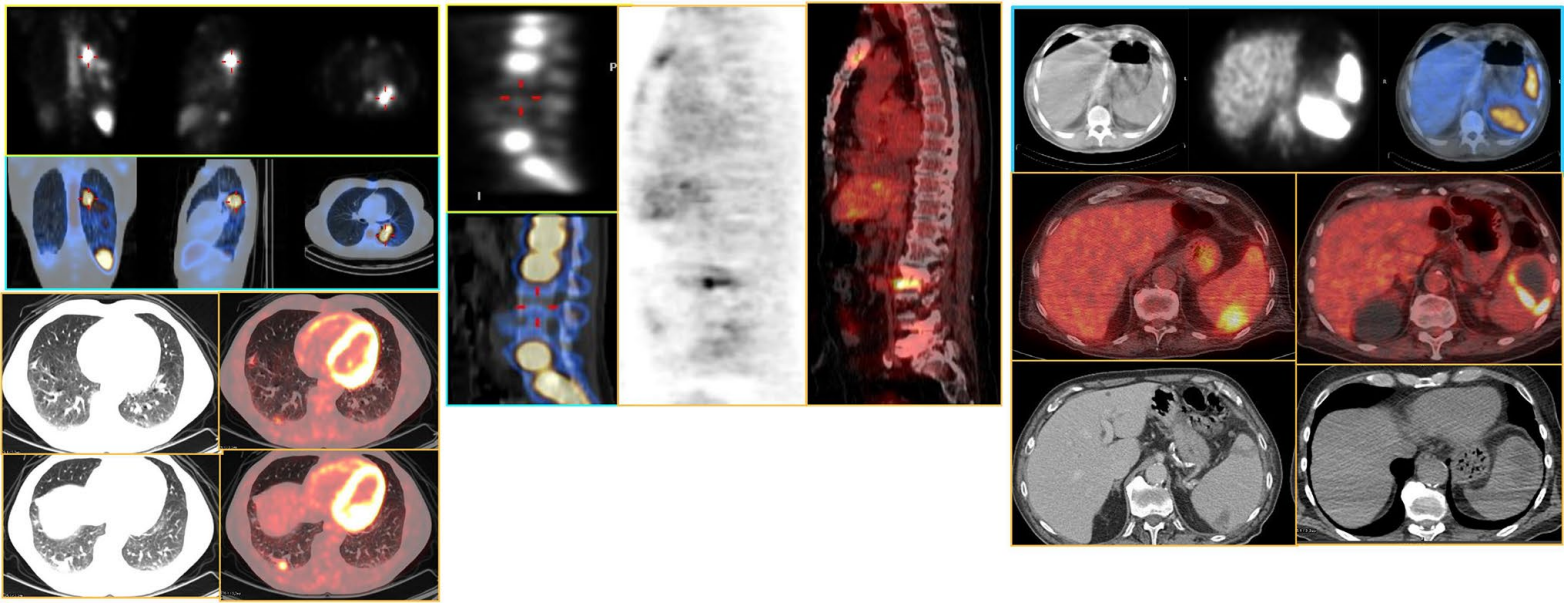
Comparison of WBC and [^18^F]FDG pattern of uptake in embolisms localized in the lung in a patient with right-sided IE appearing. In both cases, there is an area of increase radiopharmaceutical uptake (right panel WBC SPECT/CT in the upper panel in the emission and superimposed coronal, sagittal, and transaxial views, respectively, and in the lower panel [^18^F]FDG PET/CT in the transaxial CT and superimposed images at different levels). In the spine, a different pattern is seen at WBC imaging where typical cold spot is evident due to the normal high uptake of radiolabeled WBC in the normal bone marrow. On the contrary, [^18^F]FDG PET/CT finding shows increased uptake with linear pattern involving the intravertebral space, the lower aspect of the upper vertebral body, and the upper aspect of the lower vertebral body (middle panel, from right to left coronal view of WBC upper panel emission and lower panel superimposed SPECT/CT; emission and superimposed coronal [^18^F]FDG PET/CT). Embolism at spleen also presents a different uptake pattern in WBC images where photopenic area of triangular shape is often evident and [^18^F]FDG PET/CT, which can present either uptake or large cold spot (abscess) with a rim of increased uptake (left panel from top-down transaxial CT, emission and superimposed SPECT/CT of WBC, superimposed [^18^F]FDG PET/CT and CT)
